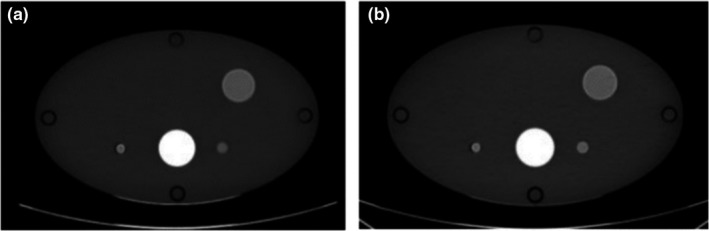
Example of images used for measuring enhancement in simple nonenhancing lesions (a) true unenhanced image (b) virtual unenhanced image reconstructed from Dual‐Energy computed tomography dataset.
